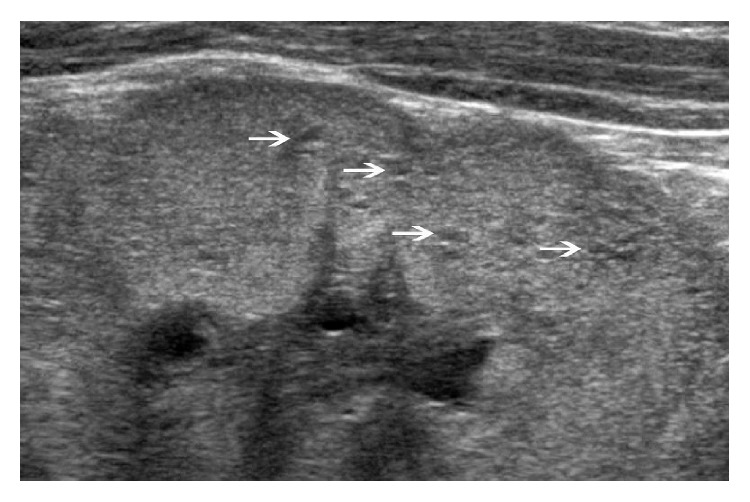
Ultrasound image of hyperechogenic kidney structure with multiple small cysts (white arrows) in the renal parenchyma.
